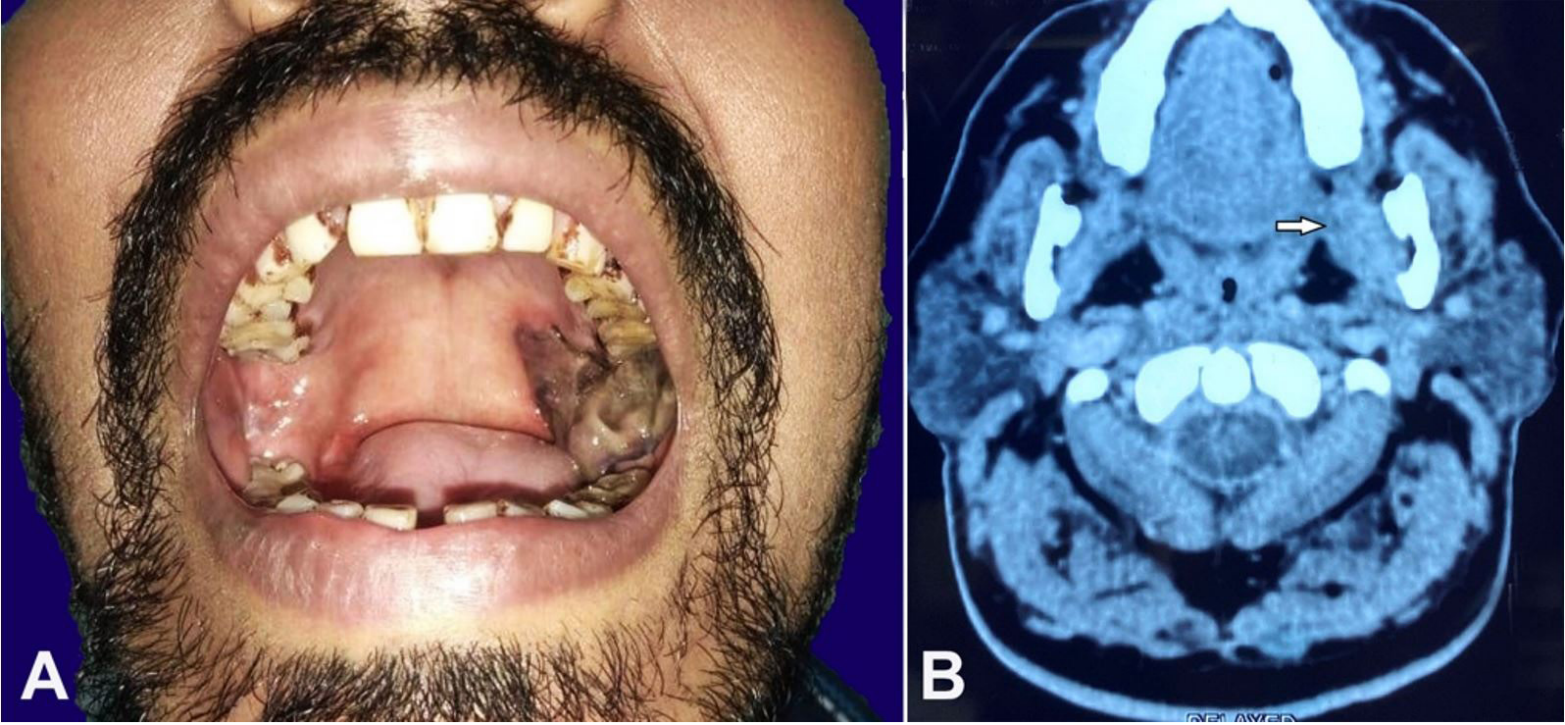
A – Tumor was found to involve the retromolar trigon (Left), extending laterally to the buccal mucosa and gingivobuccal sulcus and superiorly to the alveolar process and the hard and soft palates; B – A contrast-enhanced CT scan (Axial section) revealed a soft tissue mass in the left RMT, involving the body and ramus of mandible (left side) and the upper alveolus.
